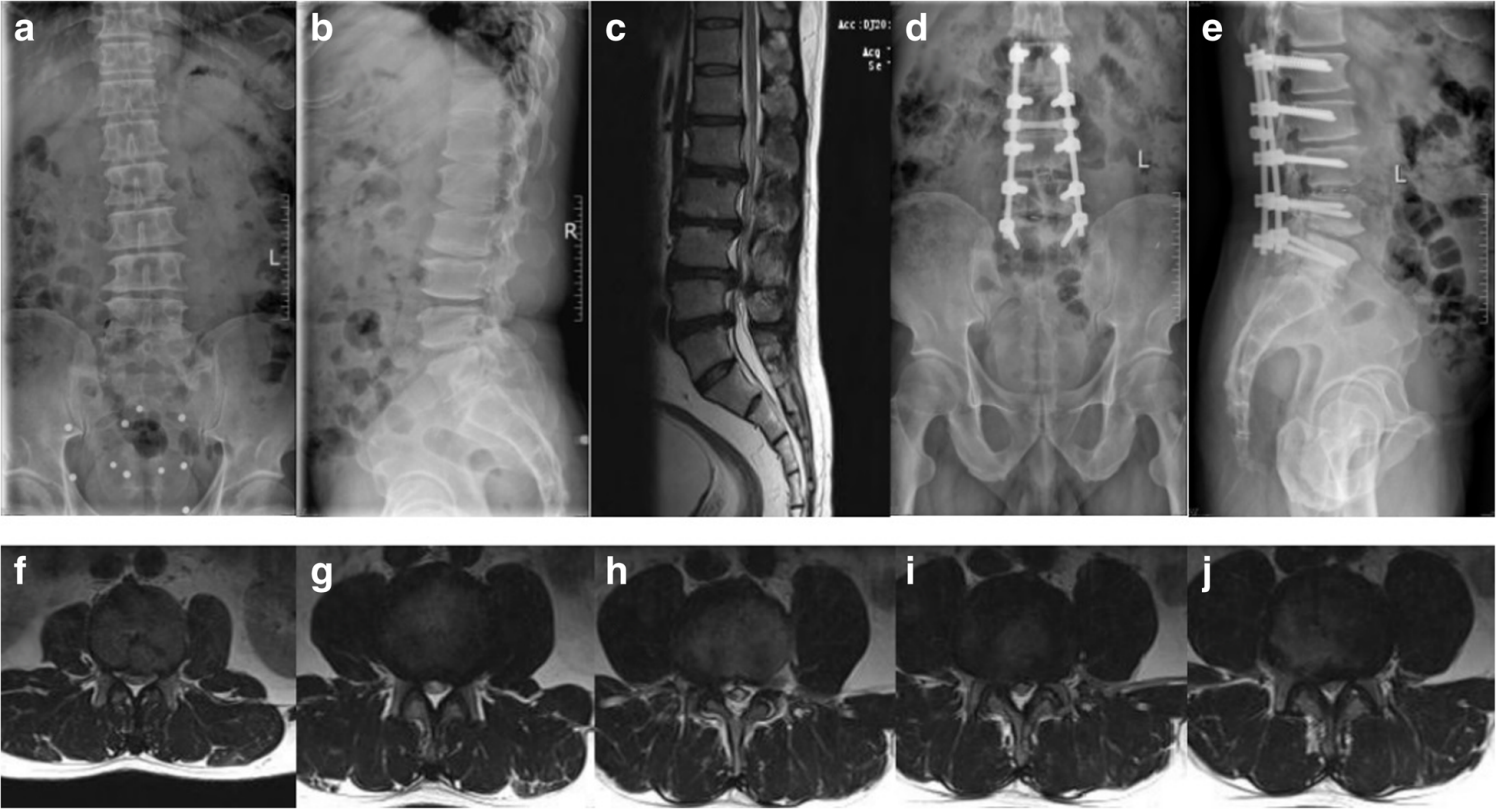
a, b Preoperative anteroposterior and lateral radiograph. c Preoperative sagittal MR image. d, e Anteroposterior and lateral radiograph 18 months after surgery showing radiographic ASD, T12/L1 disc height decreased from 7.50 to 6.0 mm. f–j Preoperative coronal MR image
